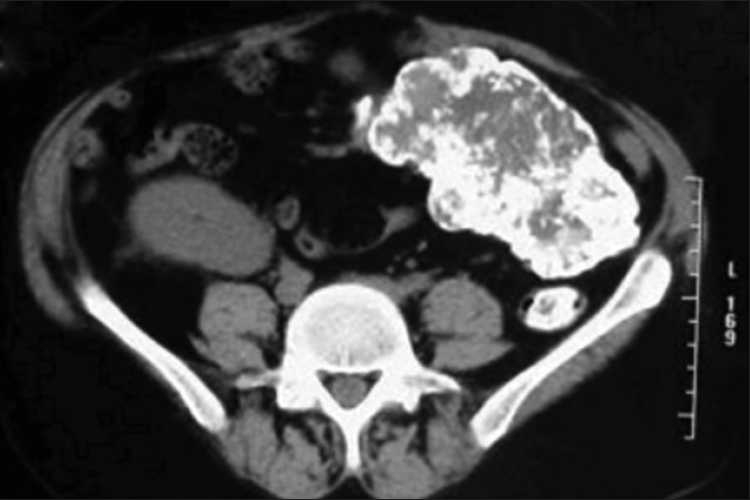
CT scan of abdomen showing, large calcified mass in left lower abdomen with extensive retroperitoneal and mesenteric lymphadenopathy
